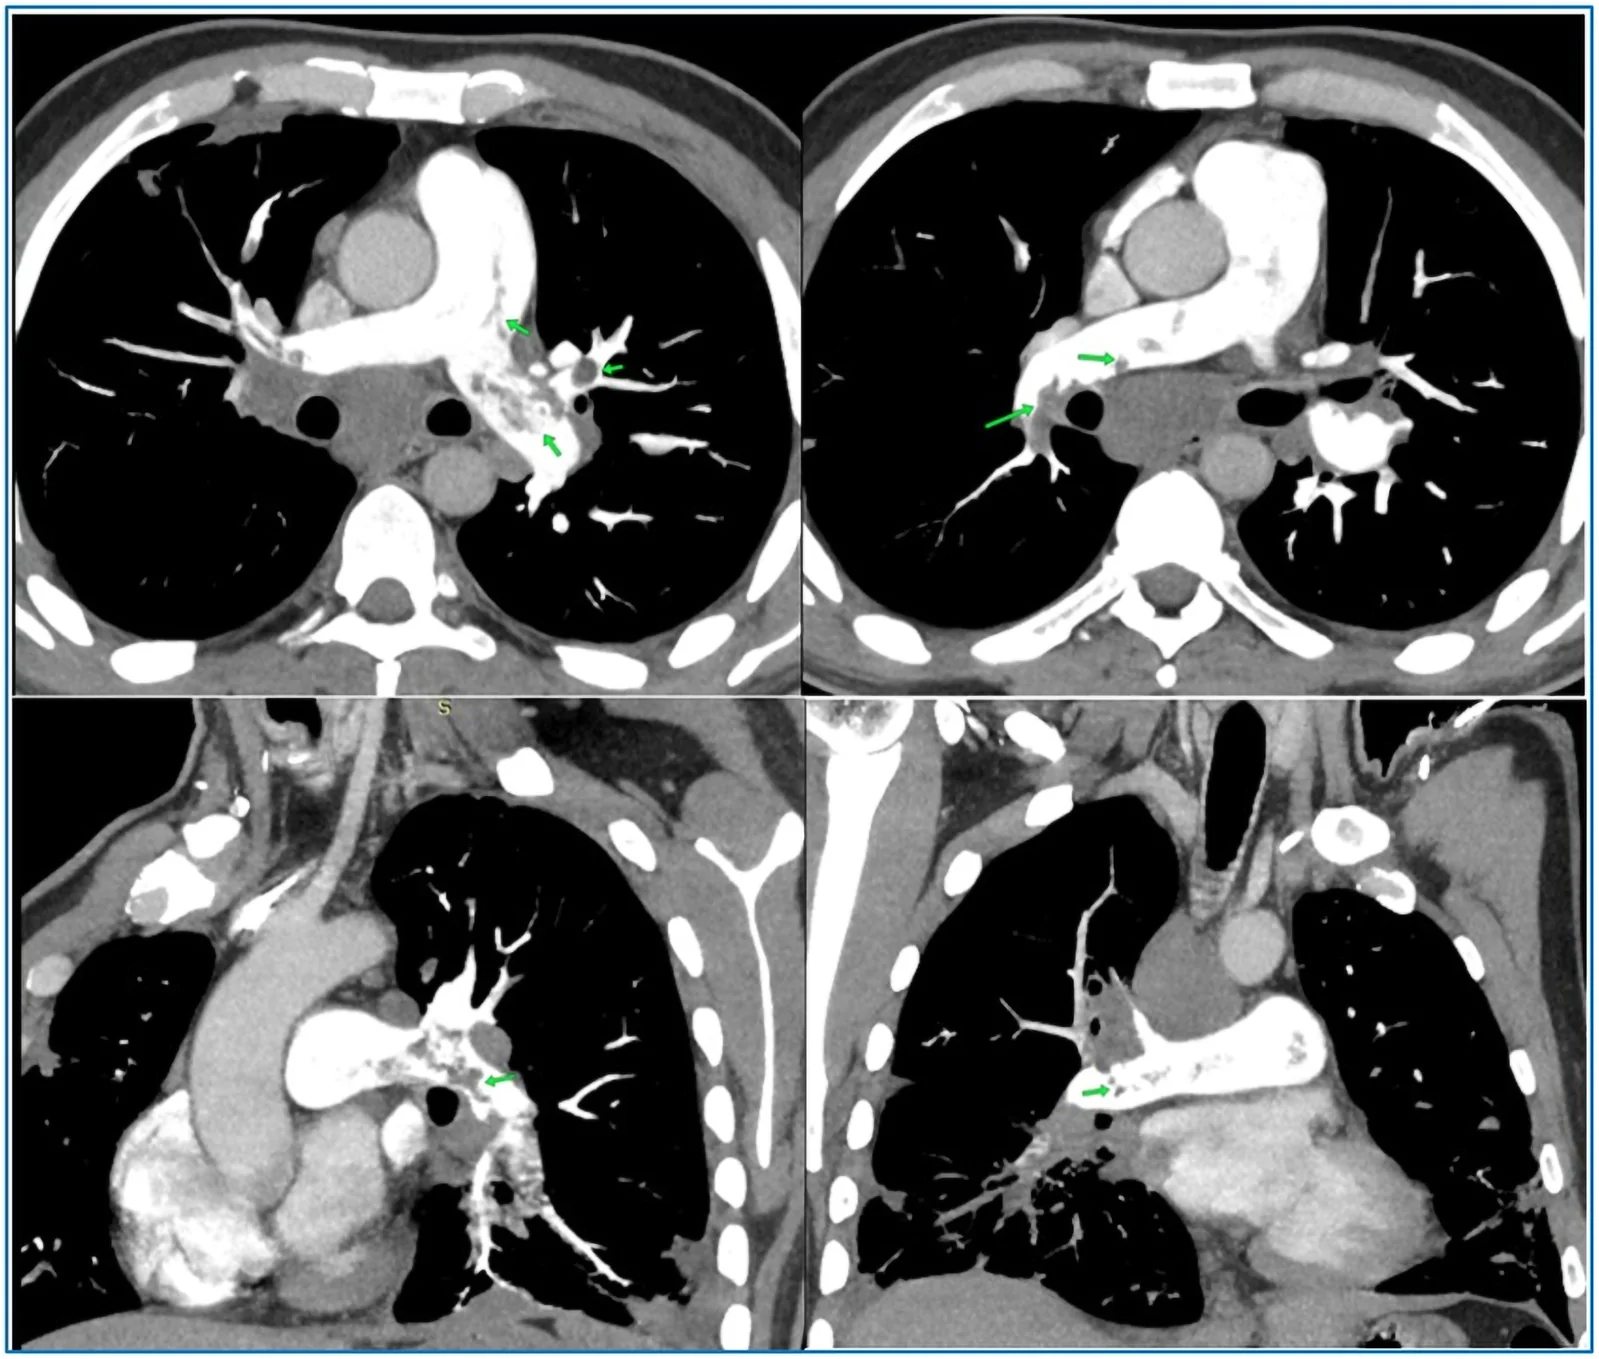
Computed tomography pulmonary angiogram. Views demonstrating filling defects and signs of chronic pulmonary thromboembolism at the main pulmonary artery bifurcation and extending into the lobar branches.
